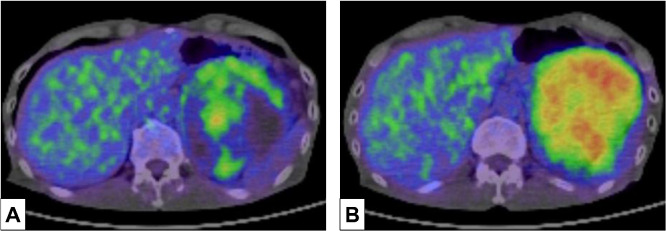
FDG PET/CT images. (A) The upper portion of the tumor. FDG uptake is observed in the soft tissue density areas of the tumor and is sparse in the fluid density areas. (B) The lower portion of the tumor. High FDG uptake is observed in the tumor.
